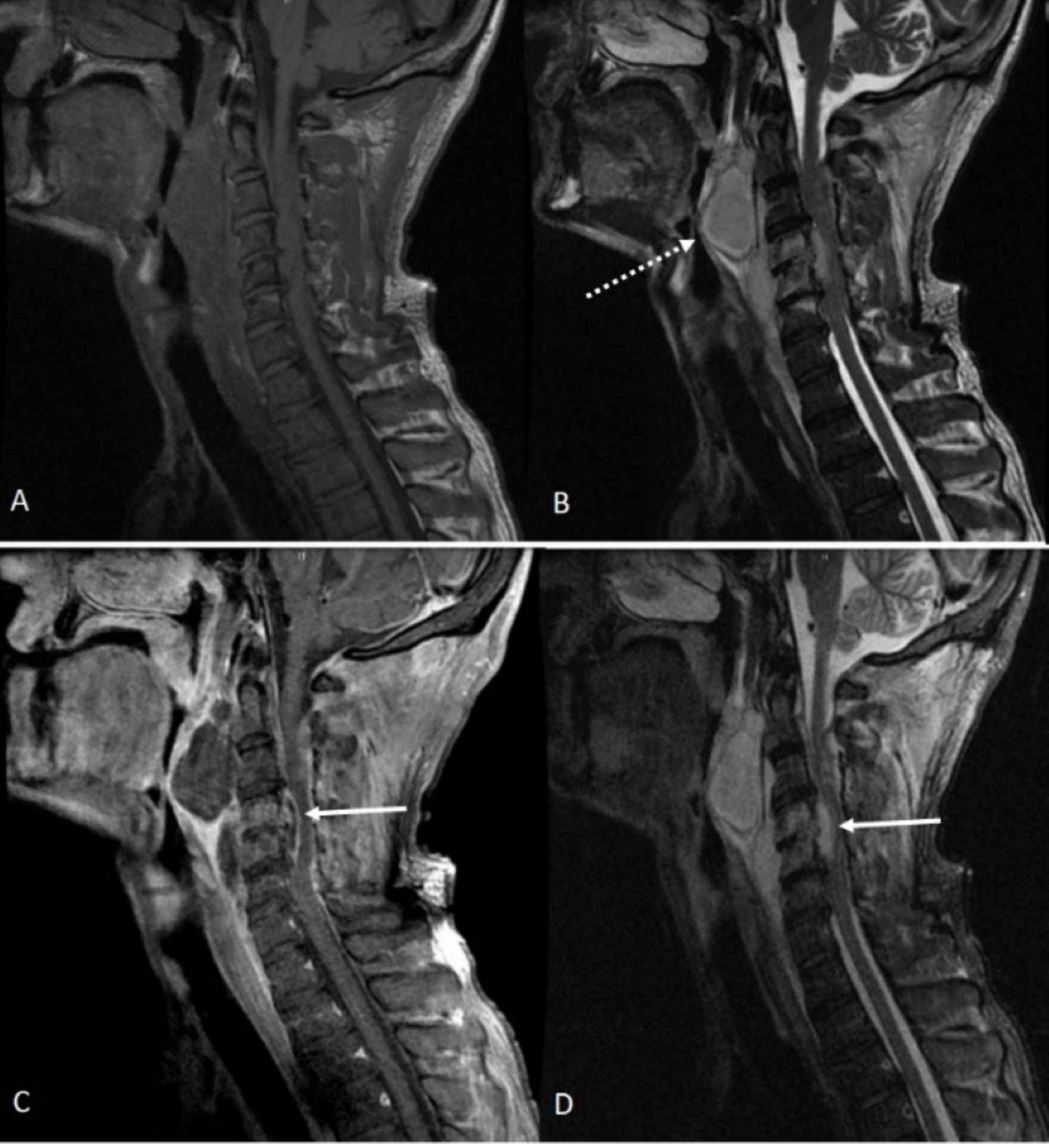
MRI of the cervical spine MRI of the cervical spine with and without contrast, sagittal sequences (A) T1, (B) T2 shows retropharyngeal abscess (dashed arrow), (C) Gadolinium-enhanced T1 shows heterogeneous enhancement in the epidural space representing an epidural abscess circumventing the spinal cord at C4-C5 (solid arrow) and hyperintensity within the C4-C5 vertebral bodies representing discitis/osteomyelitis complex, (D) short TI inversion recovery (STIR) shows epidural abscess and discitis/osteomyelitis complex at C4-C5.
